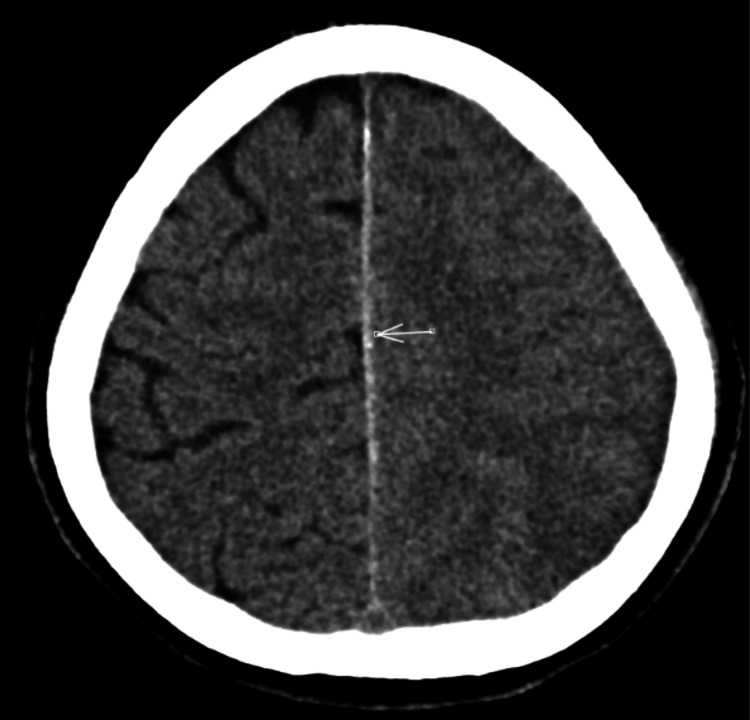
Axial non-contrast CT of the head demonstrating sulcal effacement CT of the head showing a mass effect within the left cerebral hemisphere causing sulcal effacement and a slight midline shift to the right. CT: computed tomography
